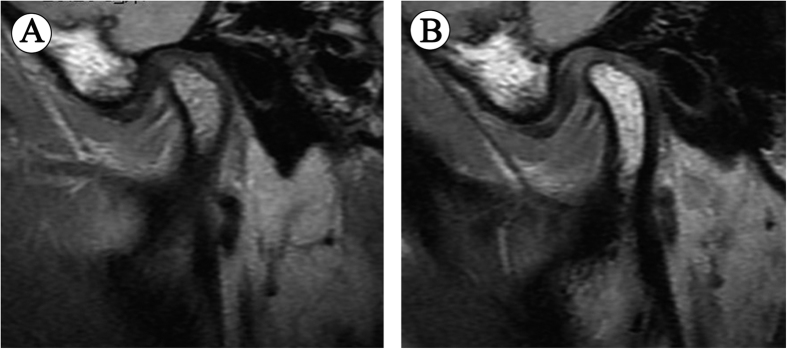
MRI scans of a 16-year-old female ADDWR patient with the interval of 21 months, showing almost unchanged disc status. (A) First visit, (B) Follow-up visit.
